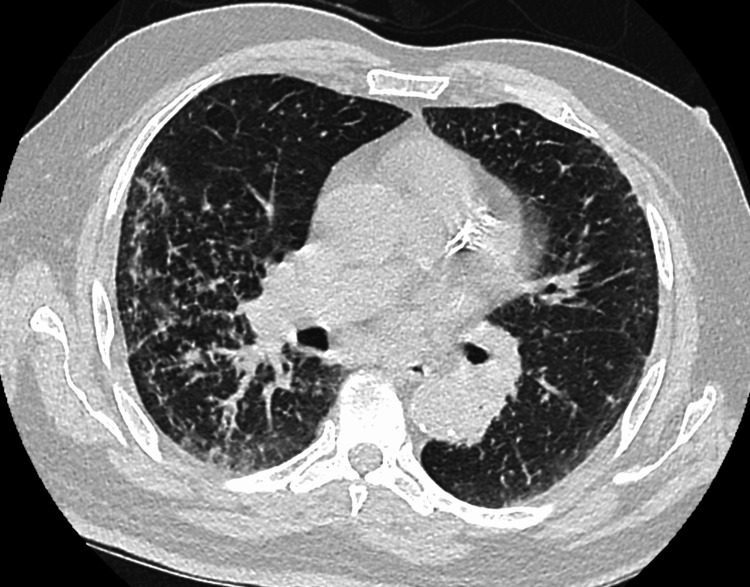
Chest computed tomography (CT) scan showing bilateral fibrotic changes in the lungs and fissure nodularity
